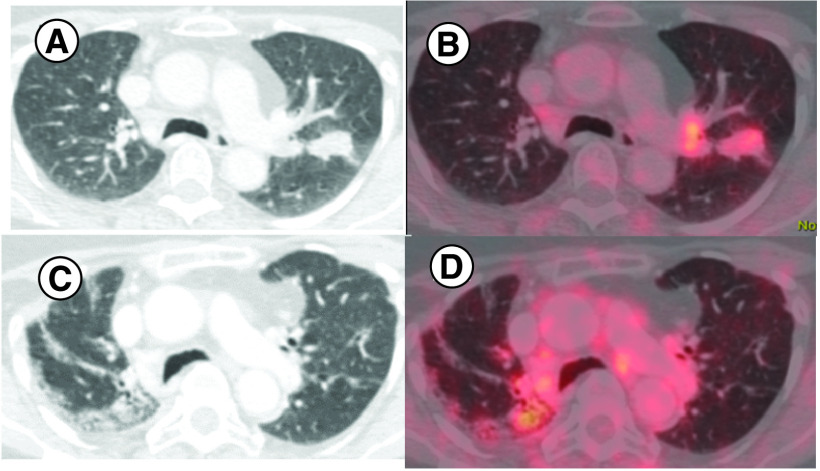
Pre treatment images of patient no.14, case of leiomyosarcoma. (A) Maximum intensity projection image of fused fluorodeoxyglucose positron emission tomography with computed tomogram (FDG PET-CT) of patient no.14 showing multiple areas of tracer uptake. (B) Axial CT images shows lytic skeletal lesions. (C) Shows increased FDG uptake in corresponding fused PET-CT. (D) Lung metastases in axial CT images. (E) Lung metastases with FDG uptake in fused PET-CT. (F) Intramuscular deposits in axial CT images. (G) FDG uptake in fused PET-CT image .
